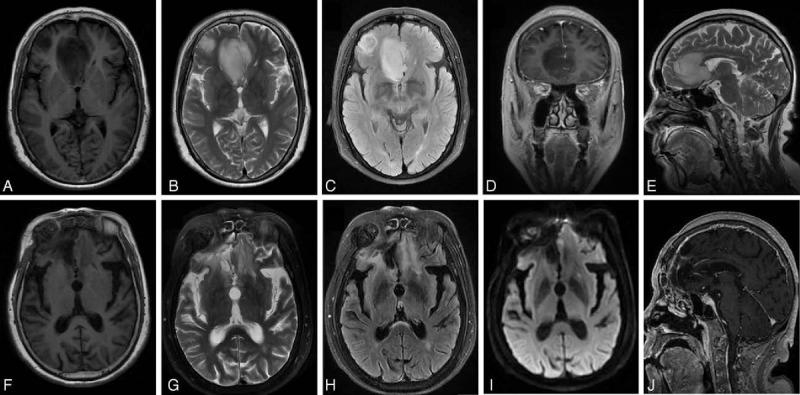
Brain magnetic resonance imaging showed a partial response of glioblastoma in a 46-year-old male patient before surgery and after 7 cycles of chemotherapy. (A) Preoperative, axial view, T1-weighed image (T1WI). (B) Preoperative, axial view, T2-weighed image (T2WI). (C) Preoperative, axial view, fluid attenuation inversion recovery image (FLAIR). (D) Preoperative, coronal view, contrast-enhanced T1WI. (E) Preoperative, sagittal view, T2WI. (F) Postoperative, axial view, T1WI. (G) Postoperative, axial view, T2WI. (H) Postoperative, axial view, FLAIR. (I) Postoperative, axial view, diffusion-weighted imaging. (J) Postoperative, sagittal view, contrast-enhanced T1WI.
